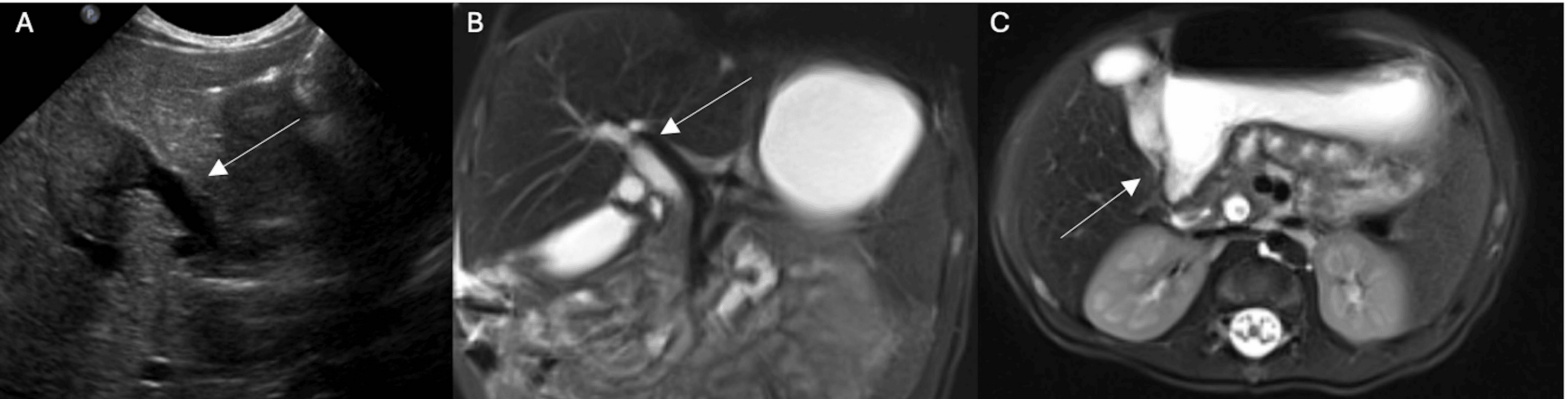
Biliary imaging for case presentation two A) Liver ultrasound showing common bile duct (CBD) and gallbladder dilation. Arrow highlights distension. B) Magnetic Resonance Cholangiopancreatography (MRCP) demonstrating dilation of the common hepatic and CBD. Arrow highlights the dilation. C) MRCP demonstrating a filling defect in the distal CBD. Arrow demonstrates filling defect.
